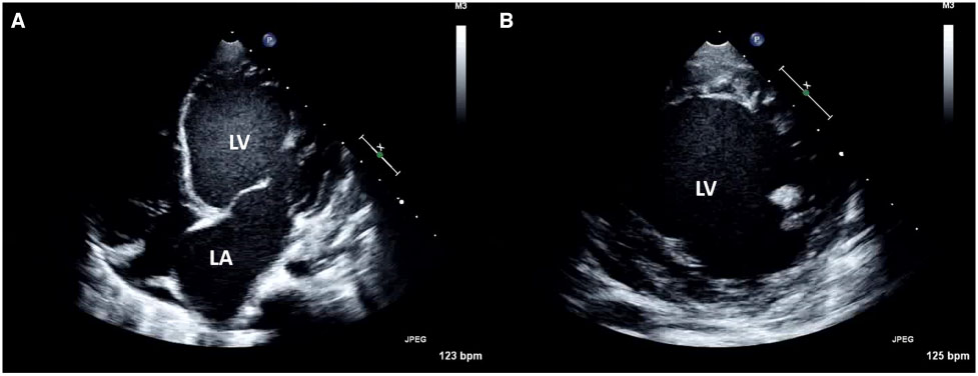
Images of echocardiography showing an extremely dilated left ventricle and atrium in (*A*) four-chamber view and (*B*) short-axis view.
